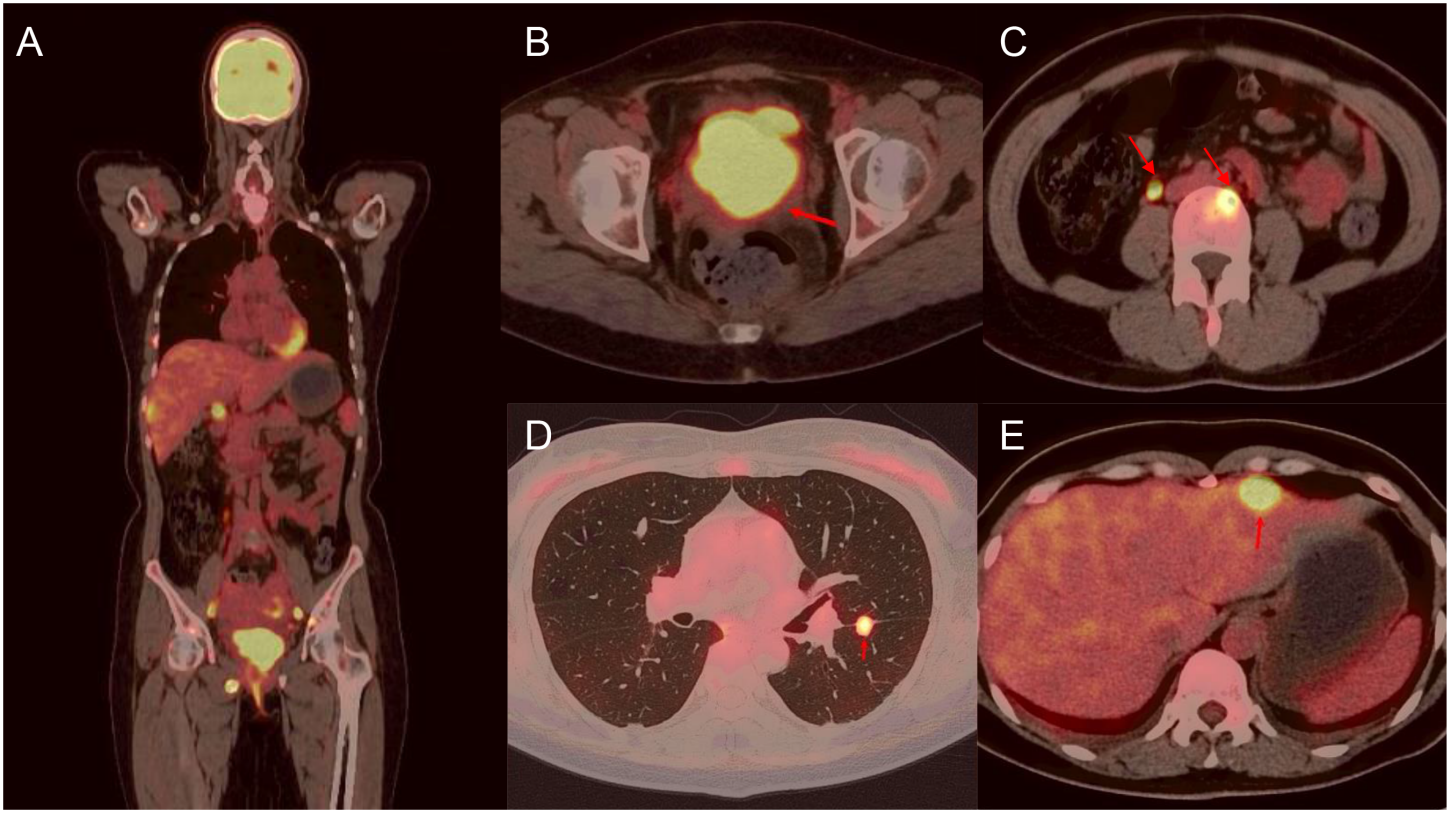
Fusion FDG-PET/CT cross section with a large lesion in the cervix and multiple metastatic lesions. There are representative images of lesions in the whole body (A), cervix (B), lumbar spine (C), lung (D), and liver (E).
